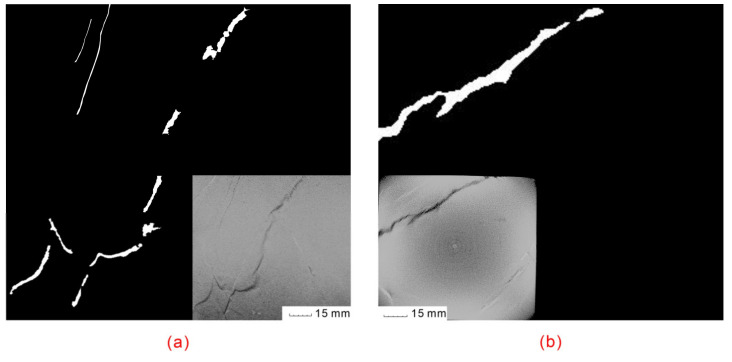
Results of microfracture segmentation and the original CT (shown in insets) after six wetting and drying cycles for sample 1. (a) At the center (z = 0 mm) of the scene coordinate system. (b) At z = 10 mm of the scene coordinate system.
